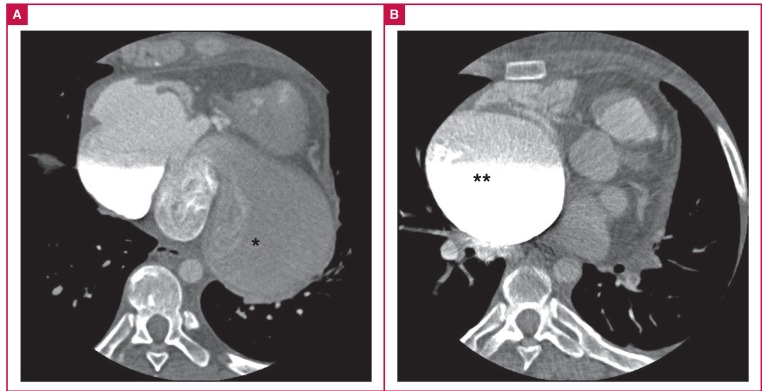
Contrast-enhanced CT showed a giant aneurysm of the coronary sinus (*) and a giant aneurysm of the superior vena cava ().
